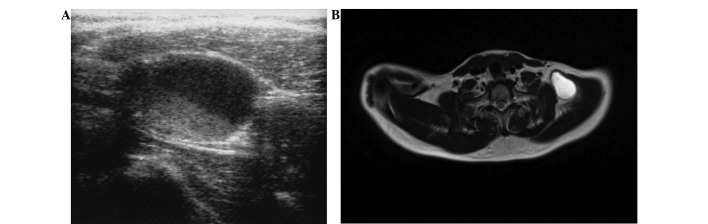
(A) Ultrasound scan showing a well-defined hypoechoic mass. (B) MRI scan showing a hyperintense lesion on the T2-weighted image.
